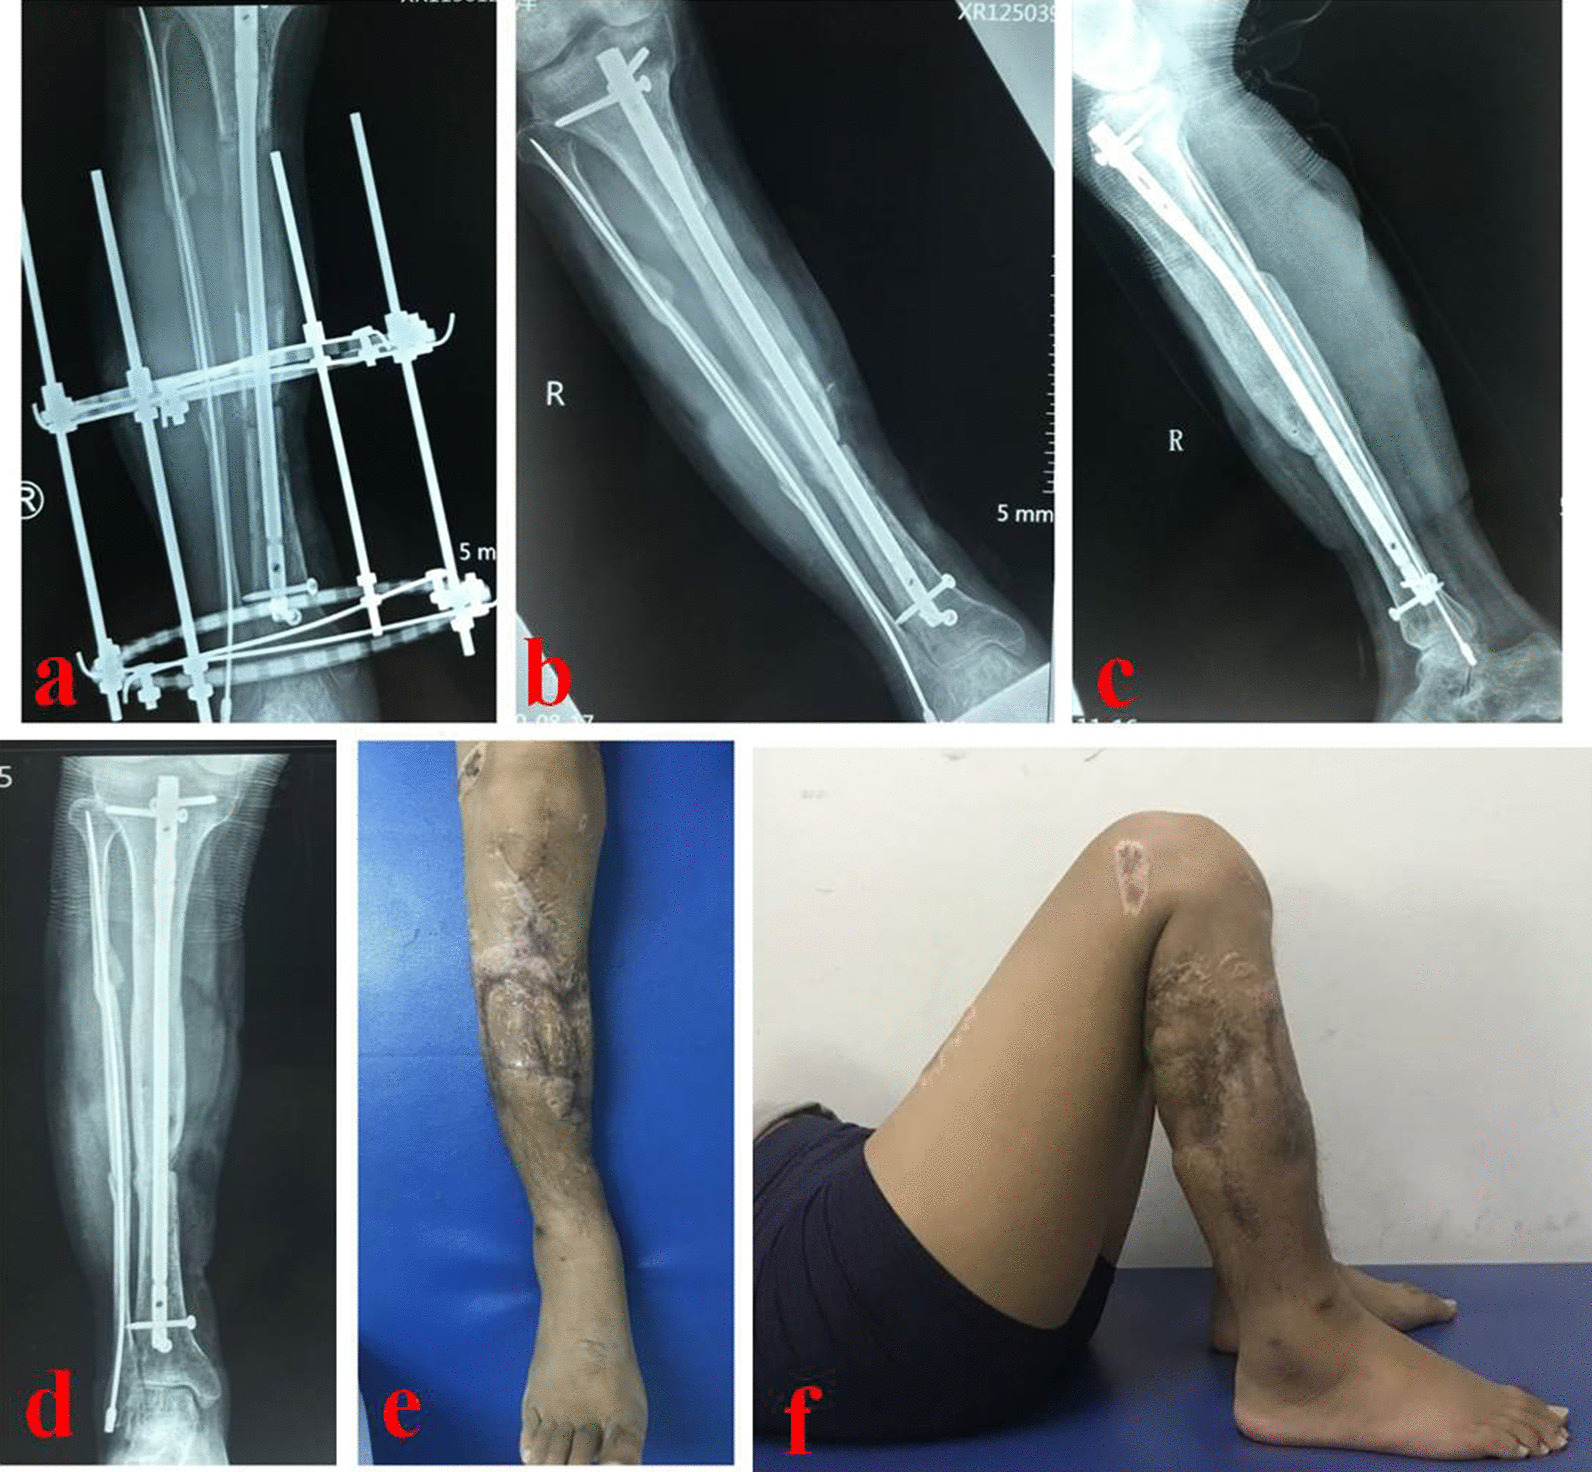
The transport process and postoperative limb functions of the 35-year-old patient. a The X-rays at 5 months showed that calcium sulfate cement was absorbed and new bones formed, b and c the external fixator was removed at 6 months after operation, and d 9 months after operation, X-rays showed that the new bone mineralization was good; e and f: postoperative appearance and knee function of the leg
